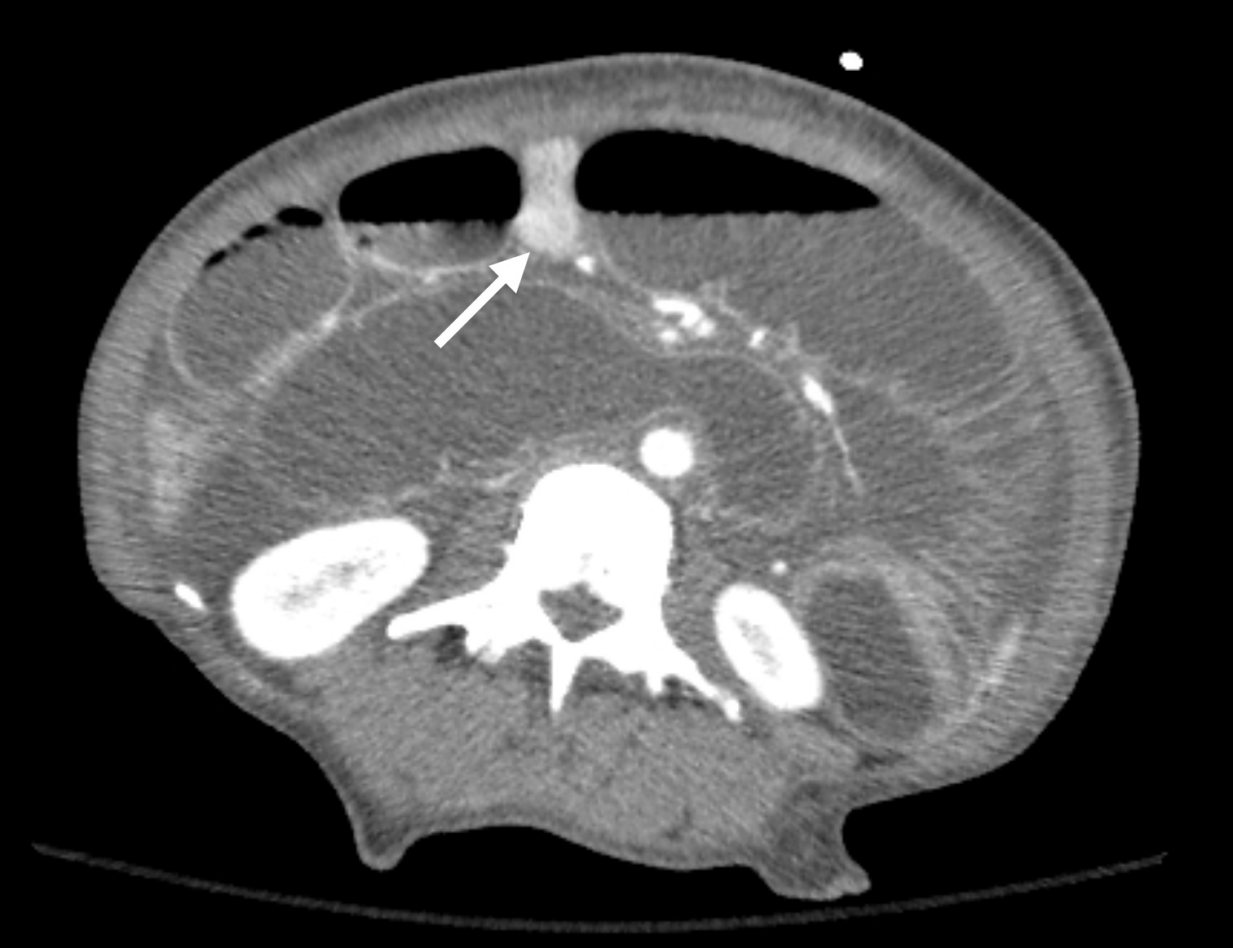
Axial CT Scan CT scan demonstrating dilated loops of the bowel and intussuscepted bowel (white arrow) at the jejunojejunostomy.
